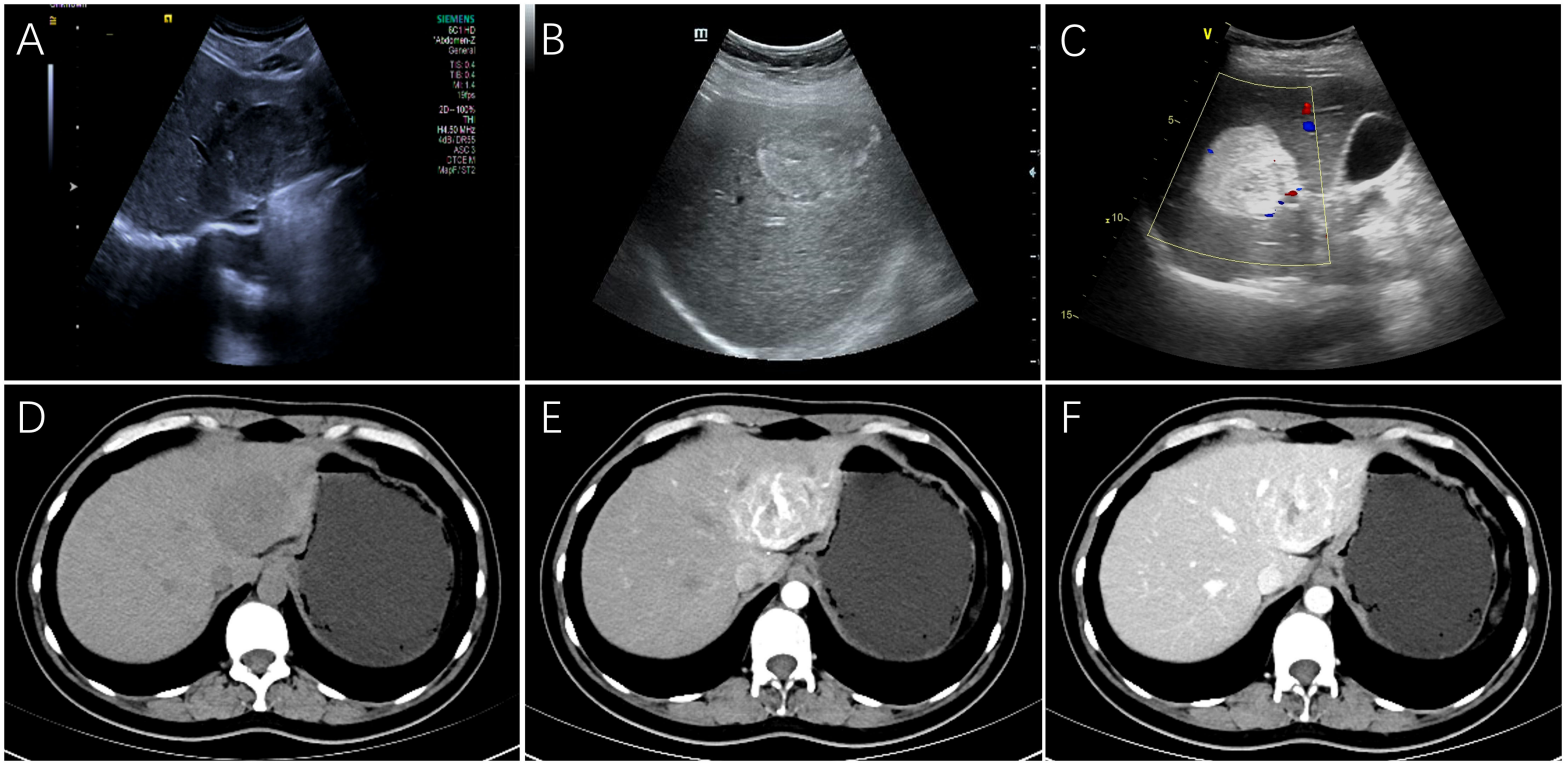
The features on Ultrasonography/dynamic CT of hepatic PEComas. Ultrasonography showed a hypoechoic mass with regular shape (A), a heterogeneous mixed echo mass (B) and a hyperechoic mass with clear boundary (C) on three different female patients, respectively. On CT scanning of a 40-year-old female patient, the plain scan showed a circular, low-density tumor with well-defined boundaries (D). Markedly inhomogeneous enhancement and abnormally dilated blood vessels can be observed in the arterial phase (E), and the enhancement decreased with washout pattern in the portal vein phase (F). (PEComa, perivascular epithelioid cell tumor; CT, computed tomography).
